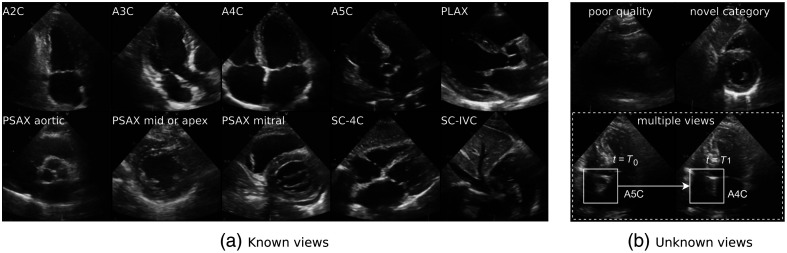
Transthoracic echocardiography view classes. (a) The known classes as part of the training set—Top row: apical two-, three-, four-, and five-chamber view; the parasternal long axis view (PLAX). Bottom row: parasternal short axis (PSAX) at the aortic valve level; PSAX at the mid-ventricle or apex; PSAX at the mitral valve; subcostal four-chamber view; the subcostal view with inferior vena cava. (b) The unknown classes to be detected while excluded from the training set—Top row: poor image quality and a novel category (here: subcostal short-axis). Bottom row: multiple views in one video.
